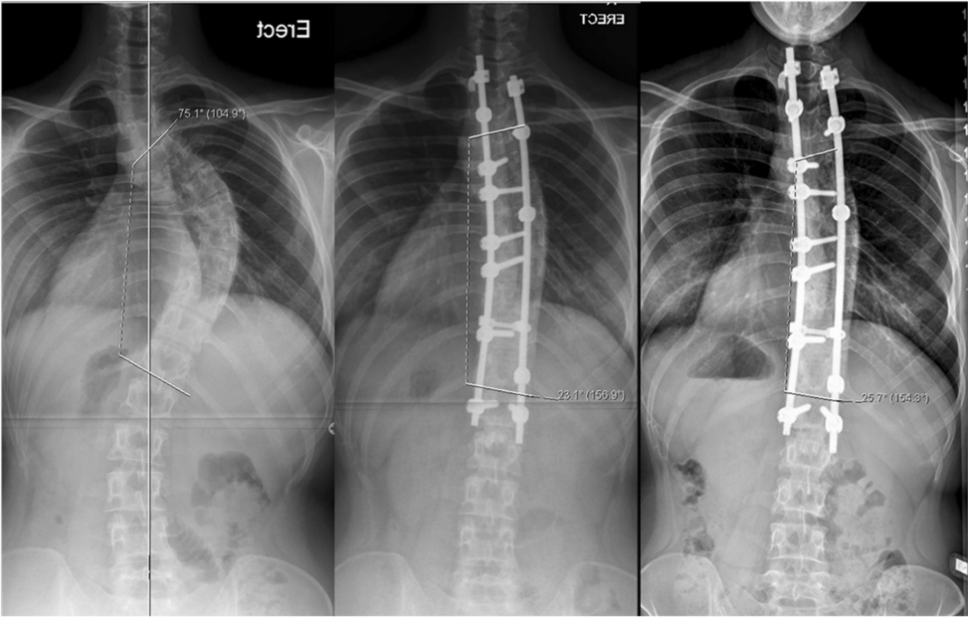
Structural thoracic AIS (*MHHN curve type I*) in 16y/♀ treated with PSF with 66 % correction showing pre-op, immediate post-op and 4 years post-op radiographs
